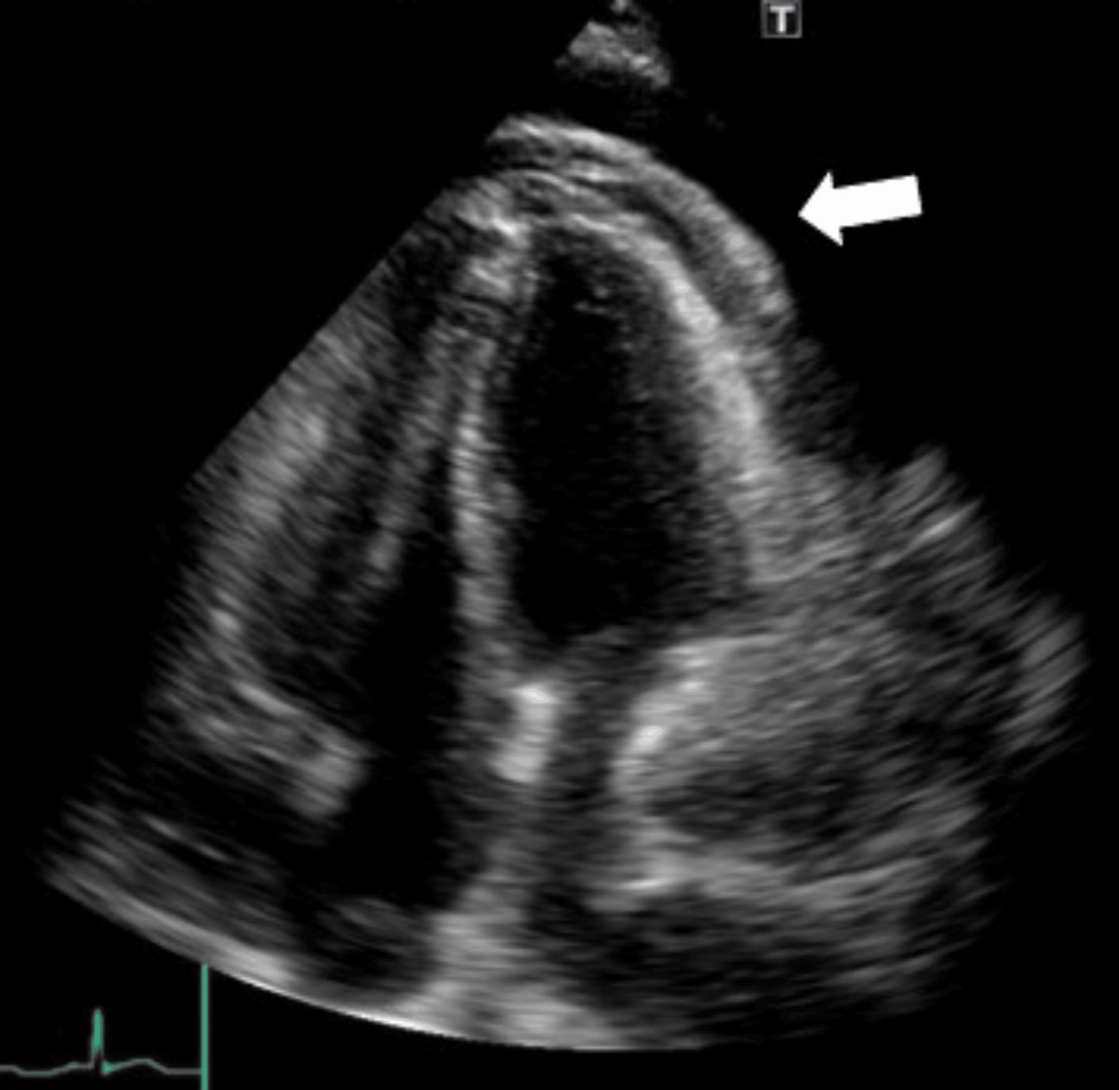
Transthoracic echocardiography after five days of admission showing increased pericardial effusion (arrow).
